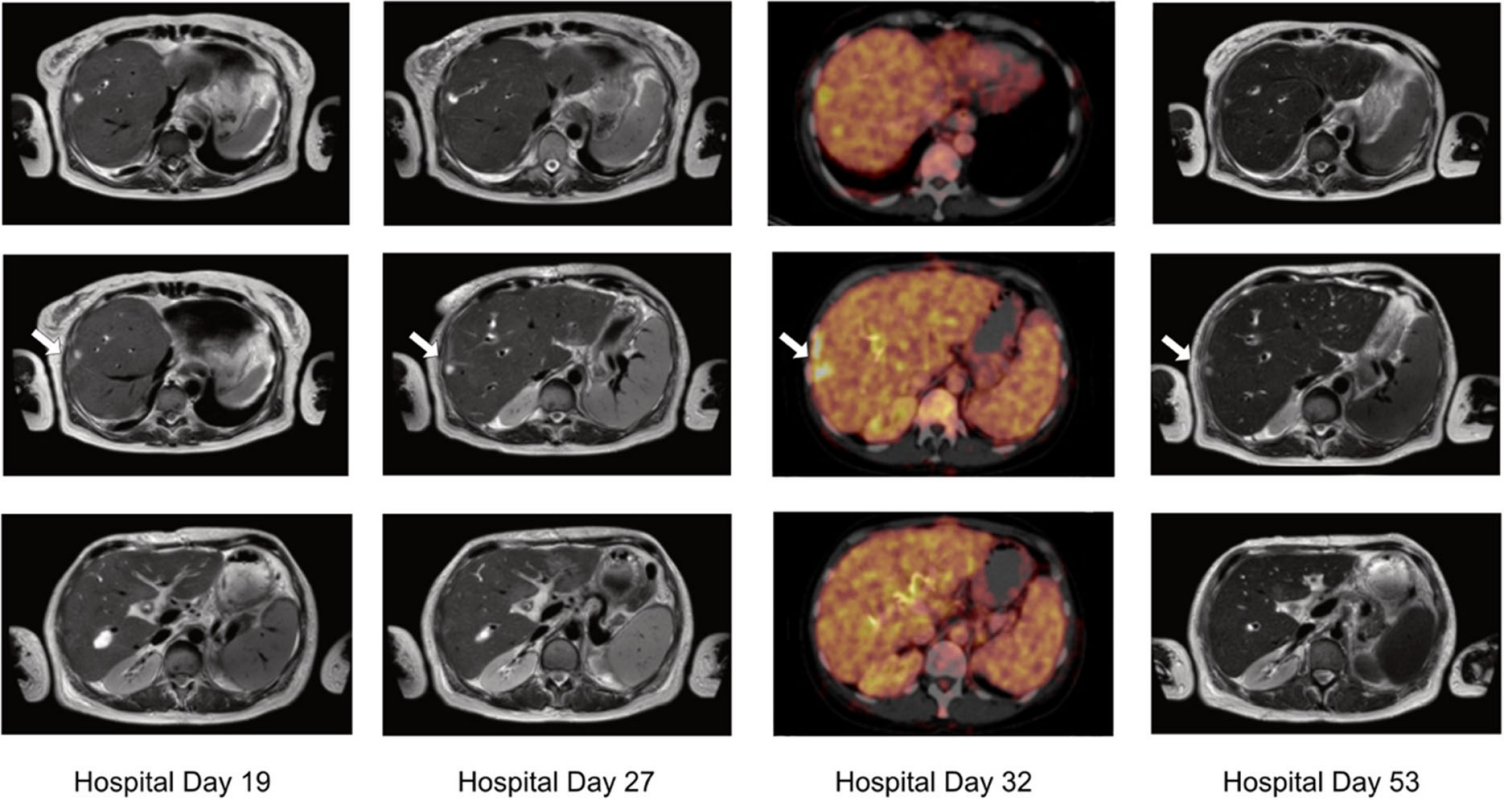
Image-based morphological monitoring of bilomas during the patient’s hospitalization at day 19 (MRI), day 27 (MRI), day 32 (PET/CT) and day 53 (MRI)
